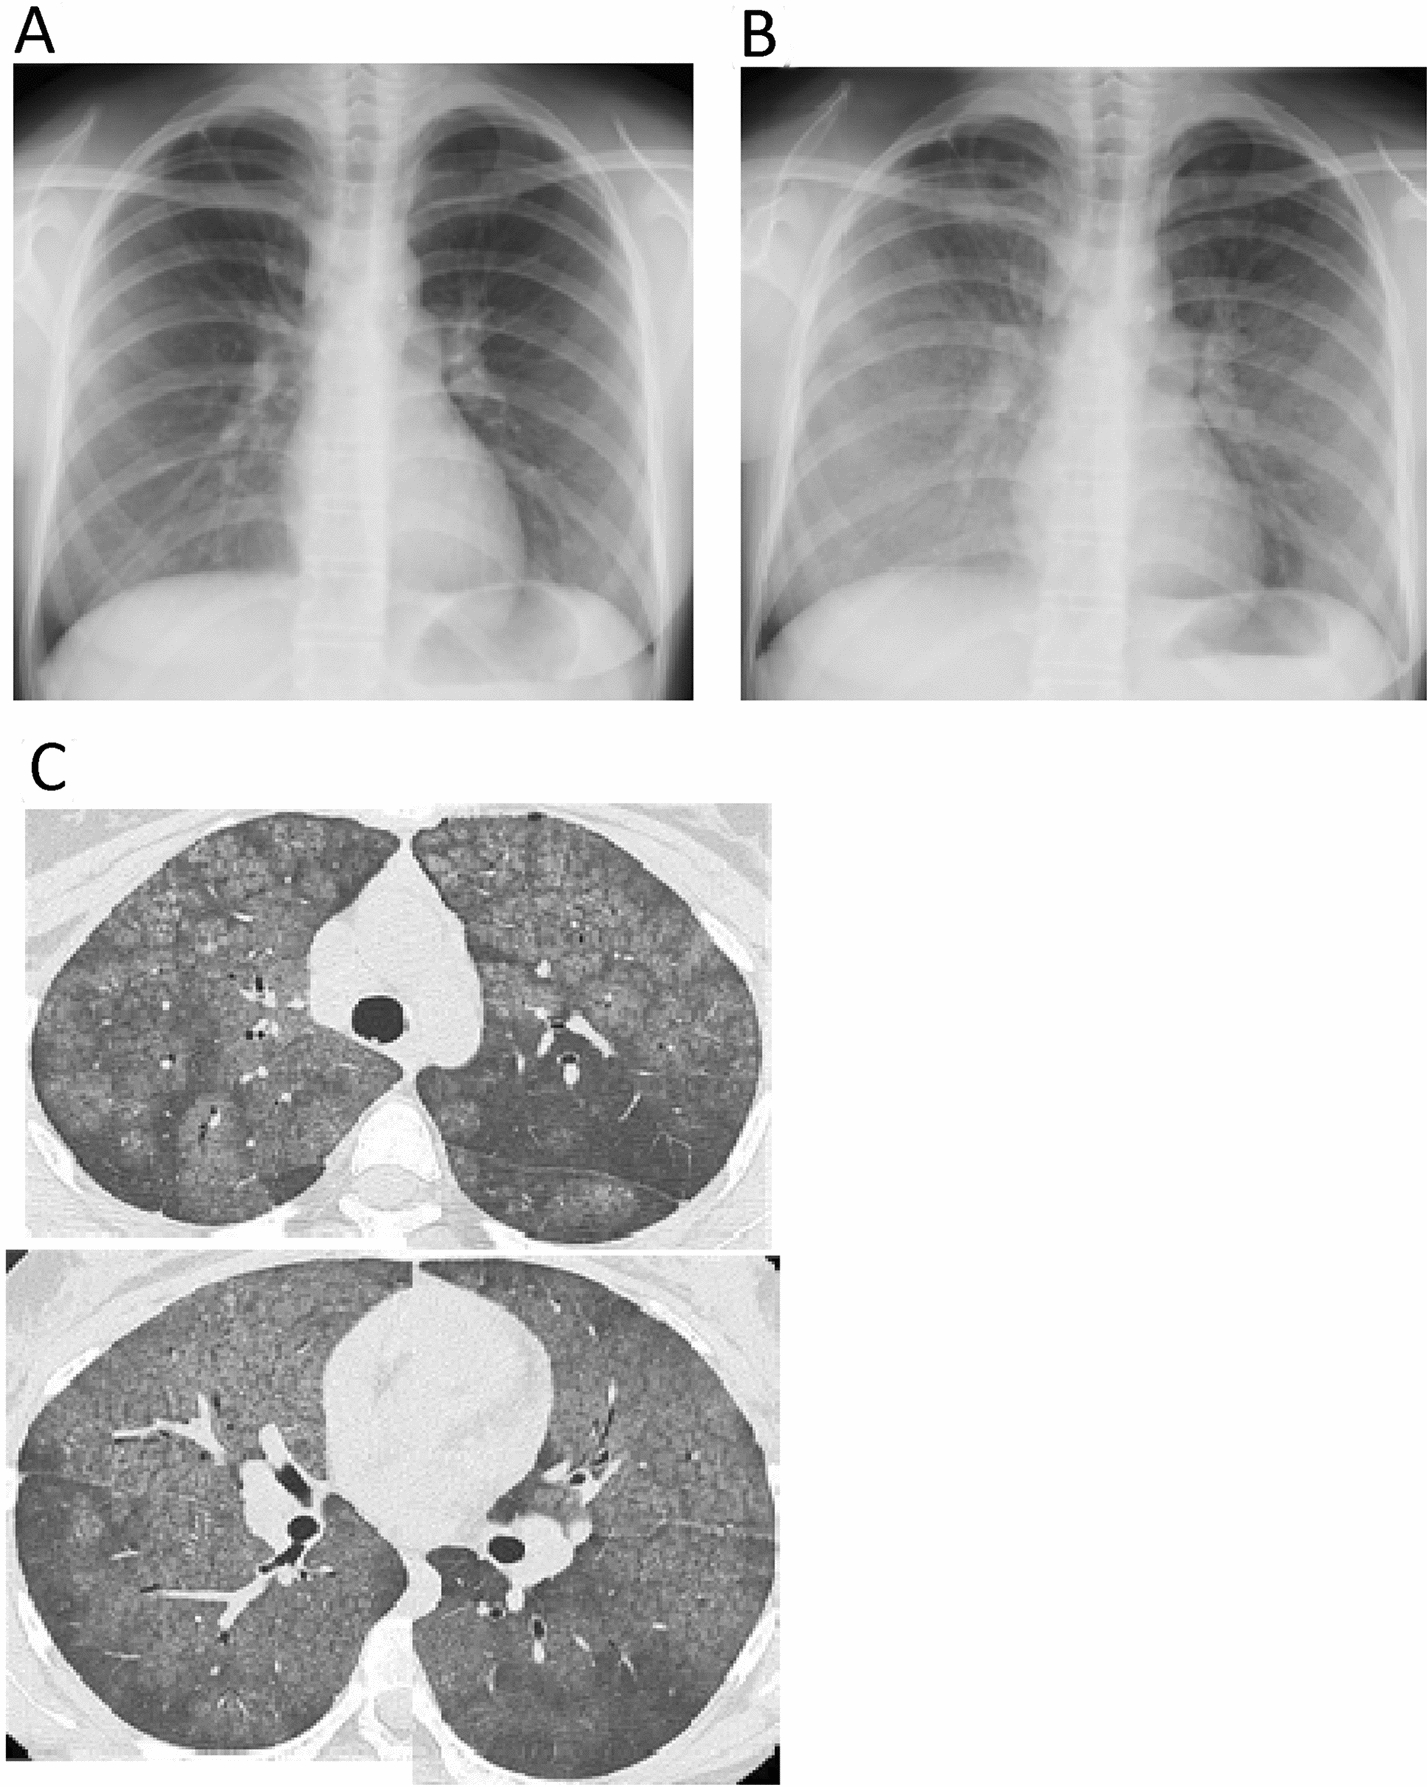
Chest X-ray images and chest CT image after tocilizumab re-administration. Chest X-ray images 8 (A) and 11 (B) days after tocilizumab re-administration and chest CT images 11 days after tocilizumab re-administration (C)
